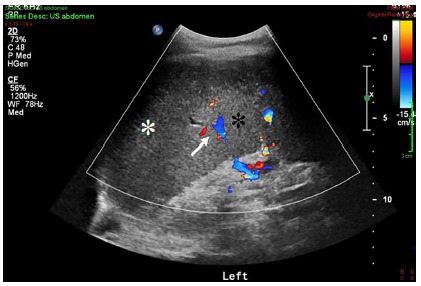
Longitudinal color Doppler scan of the spleen demonstrating an upper
